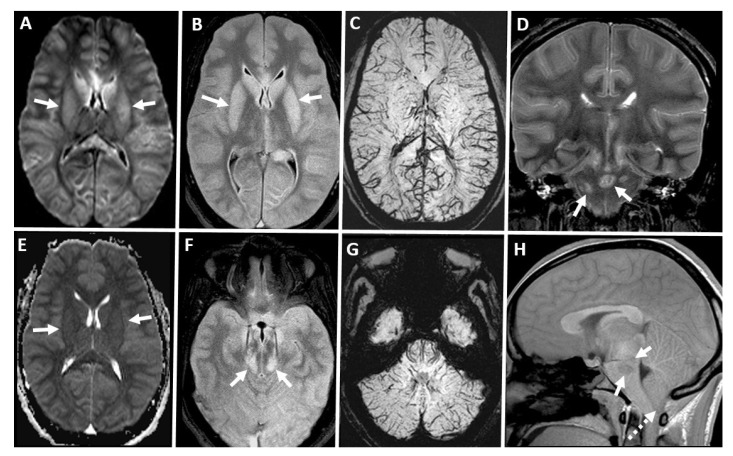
(A) Axial diffusion-weighted (repetition time msec/echo time msec, 8500/100; flip angle, 90°; b value = 1000 sec/mm^2^) and (E) corresponding axial apparent diffusion coefficient maps (8500/100; flip angle, 90°; b value = 1000 sec/mm^2^). (B,F) Axial FLAIR (fluid-attenuated inversion recovery; inversion time 2500, repetition time msec/echo time msec, 9000/107, 5 mm section thickness), (C,G) axial susceptibility weighted (repetition time msec/echo time msec, 28/20, 1.5 mm section thickness), (D) coronal T2 weighted (repetition time msec/echo time msec, 3711.1/93, 4 mm section thickness), and (H) sagittal T1-weighted unenhanced (repetition time msec/echo time msec, 250/2.48, 5 mm section thickness). MRI images of the brain in a patient with BD/DNC. There is restricted diffusion with FLAIR high signals in bilateral caudate and putamen deep nuclei (solid white arrows in (A,B,E)) with increased cortical SWI venous signals in supra and infratentorial brain parenchyma (C,D). Associated descending tentorial and tonsillar herniation (dashed white arrow in (H)) with durate hemorrhages in bilateral mid brain and pons (solid white arrows in (D,F,H)).
